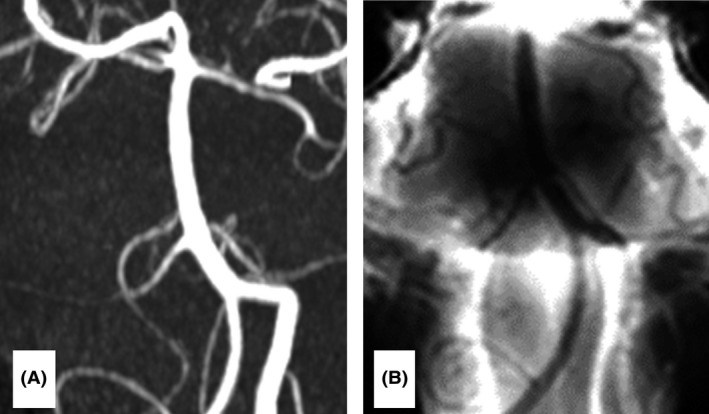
Repeat magnetic resonance angiography (A) and basi‐parallel anatomical scanning–magnetic resonance imaging (B) showing complete resolution of the vasoconstriction in a 41‐year‐old man with reversible cerebral vasoconstriction syndrome of the basilar artery.
